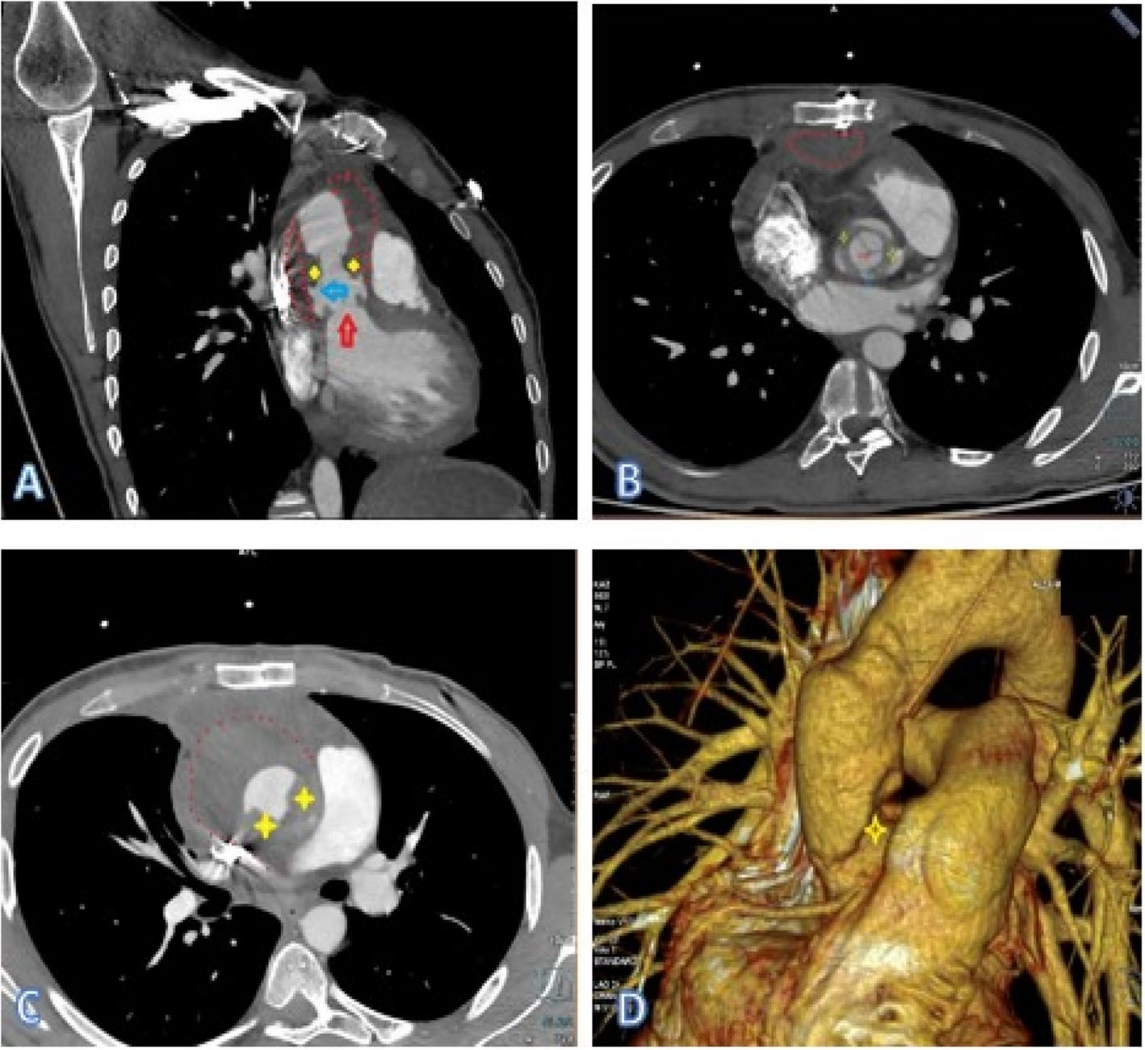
A, Oblique coronal reformatted; B, axial oblique reformatted at the aortic valve level; C, axial oblique reformatted; and D, Volume rendering reconstruction of Aortic CT angiography depicts disruption (blue arrow) of aortic root just above the valve (red arrow) along with a large pseudoaneurysm (red dots). An Apple core-like appearance (yellow four-point stars) due to vegetation is seen in the mid-ascending aorta. Most of the pseudoaneurysm is not opacified as it is full of vegetation
